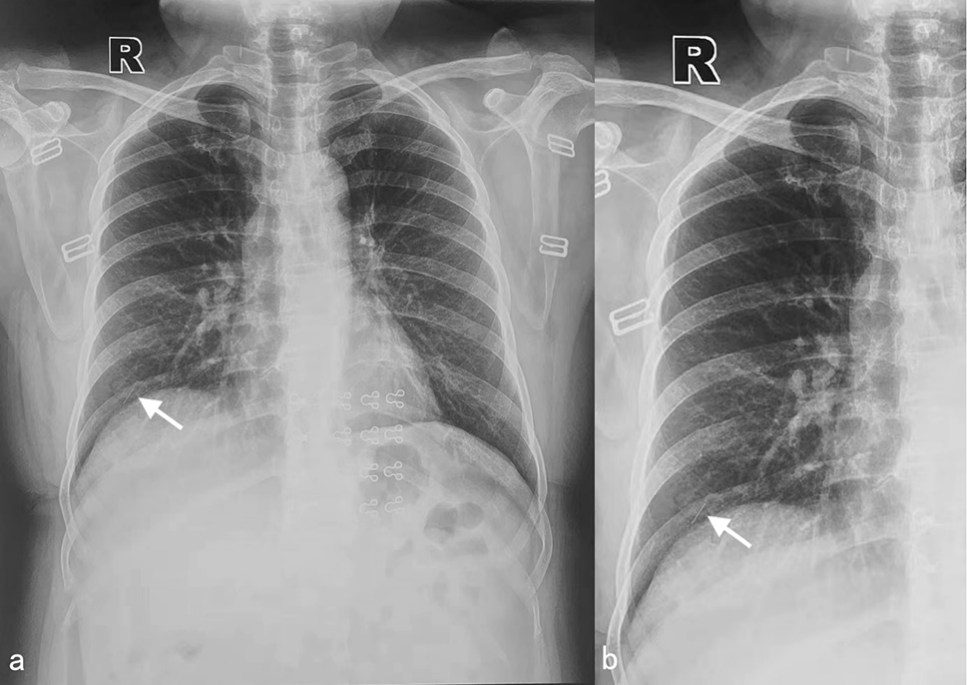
Preoperative X-ray examination: (a) isometric; (b) enlarged
